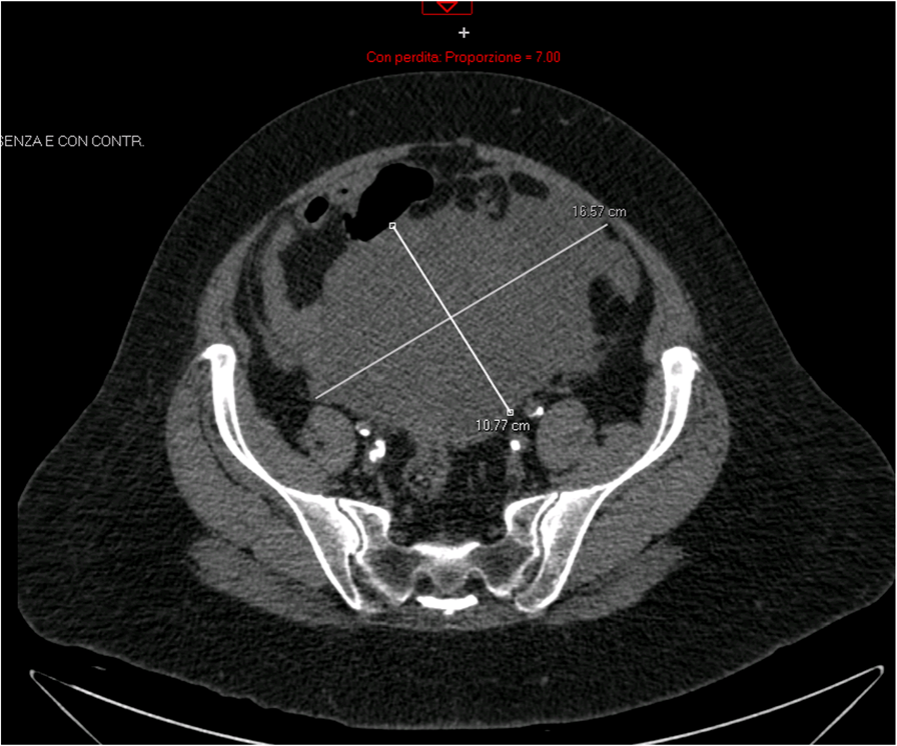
Computed tomography scan showing a mass of 16 × 11 cm
